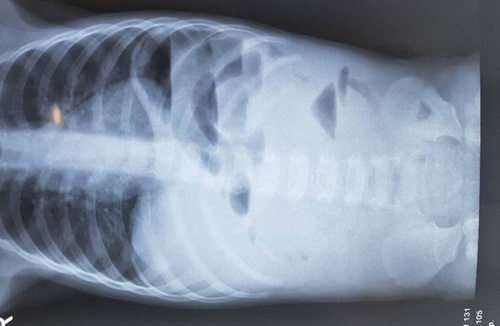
Third abdominal X-ray showed the same clinical picture.
